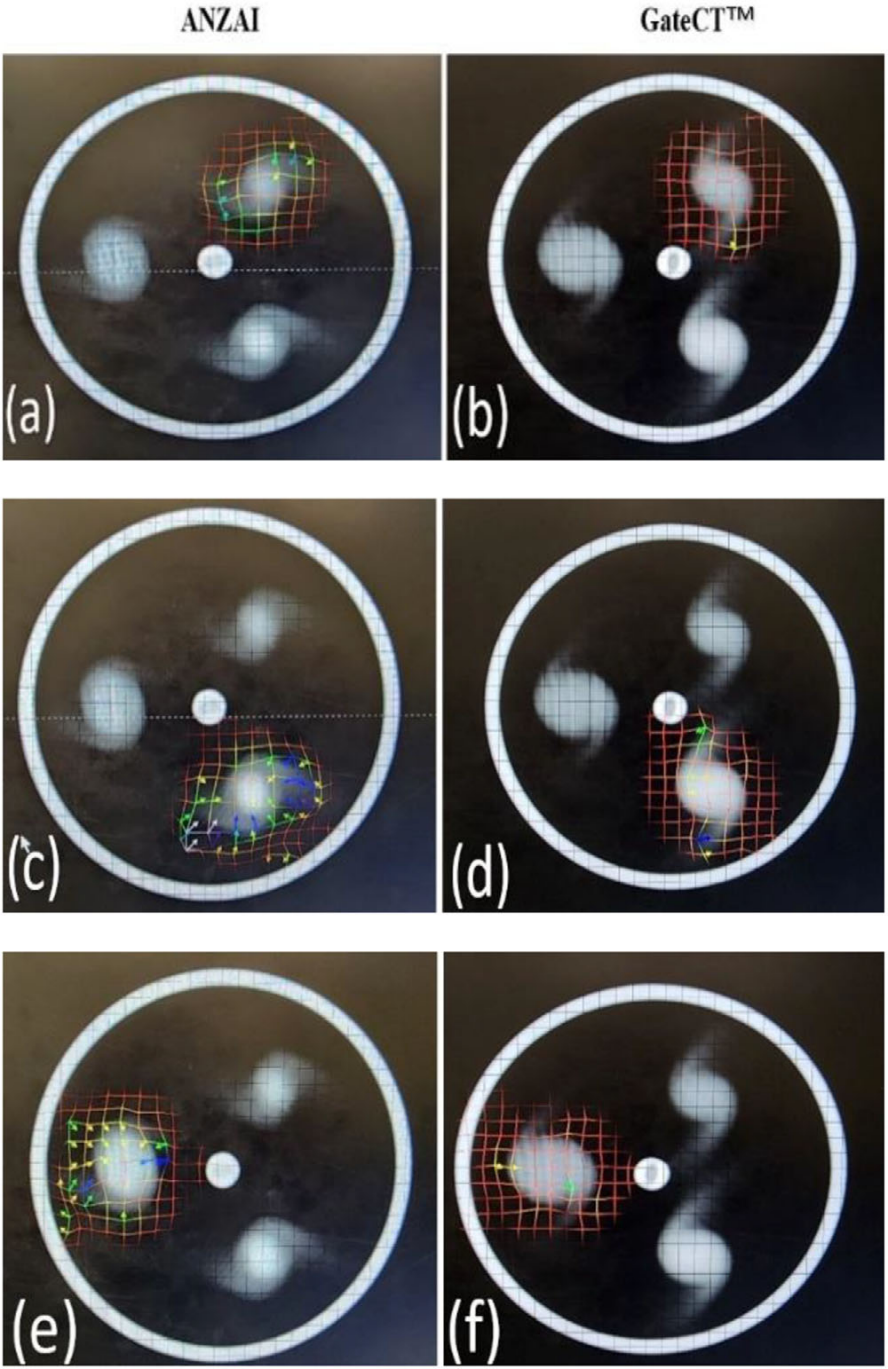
Deformation grid of the three spheres with respect to the static CT at 100% inhale phase for ANZAI versus GateCT systems. Deformation vector fields are depicted for: (a) and (b) sphere A, (c) and (d) sphere P, and (e) and (f) sphere R deformation.
